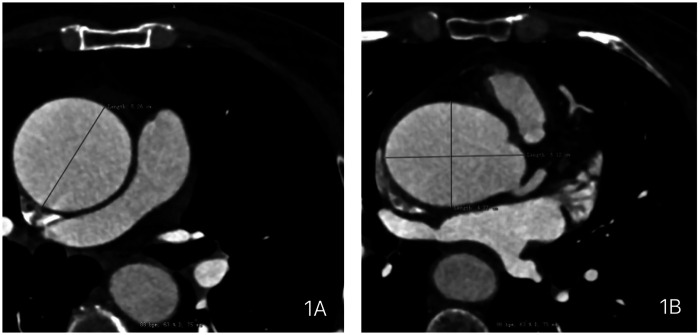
Preoperative chest computed tomography (CT) of the patient demonstrating: (A) dilated ascending aorta with a maximum diameter of 5.26 cm. (B) Dilated aortic sinus with dimensions of 6.12 × 4.77 cm.
